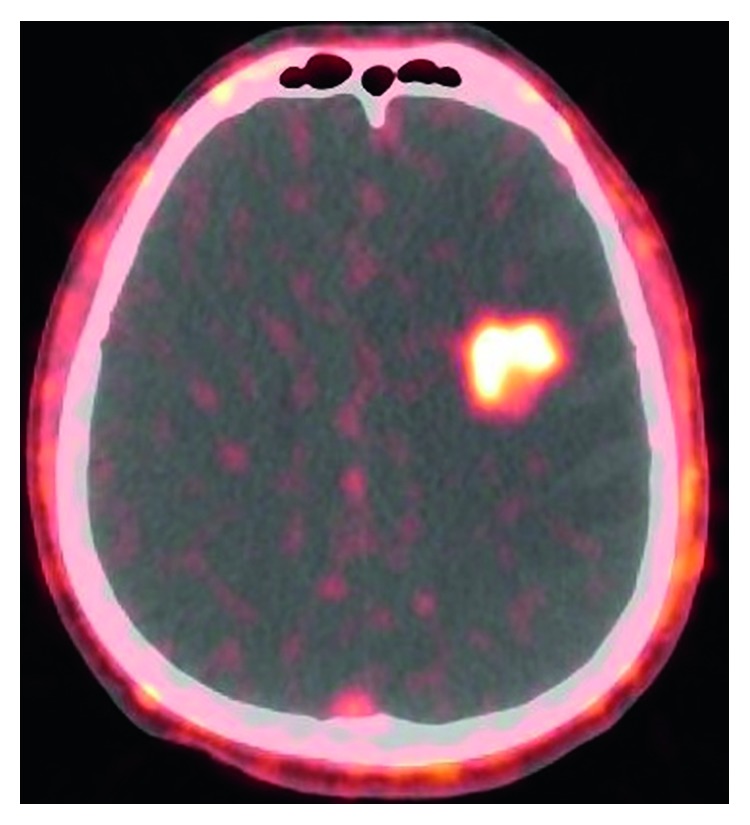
Representative PET/CT [18F]ML-10 image of a male patient, 48 years old, diagnosed with kidney cancer brain metastases, pre-CK.
